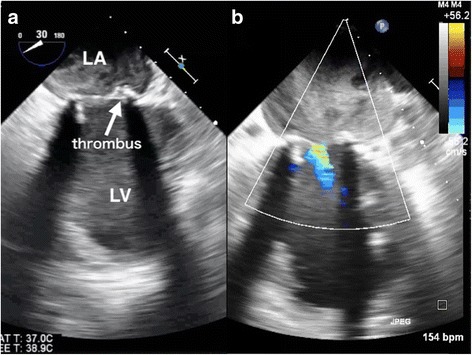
a Immobilization of the bioprosthetic mitral valve due to thrombus was observed. Left atrial spontaneous echo contrast was also seen. b The thrombus was obstructing transmitral inflow, as seen in the aliased flow pattern using color flow Doppler. *LA* left atrium, *LV* left ventricle
